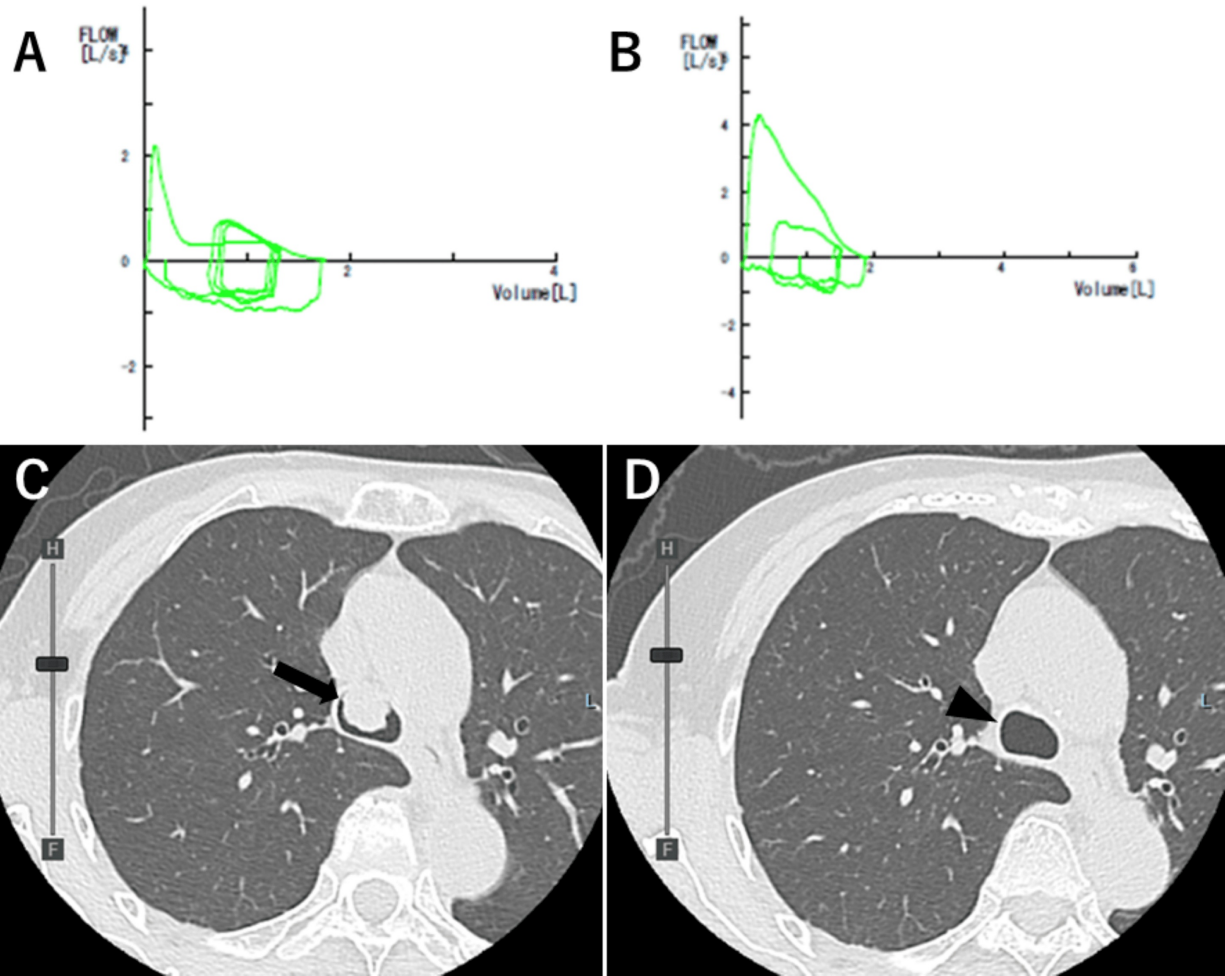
Pulmonary function and images before and after tracheal tumor removal (A) Pre-treatment flow-volume curve showing severe obstruction, with a flattened expiratory limb indicative of fixed upper airway obstruction. (B) Post-treatment flow-volume curve demonstrating significant improvement in airflow, with a more normal curve shape and increased peak expiratory flow rate. (C) Pre-treatment and (D) four months after treatment of chest CT images. The large tumor obstructing most of the trachea (black arrow) was removed (black triangle).
